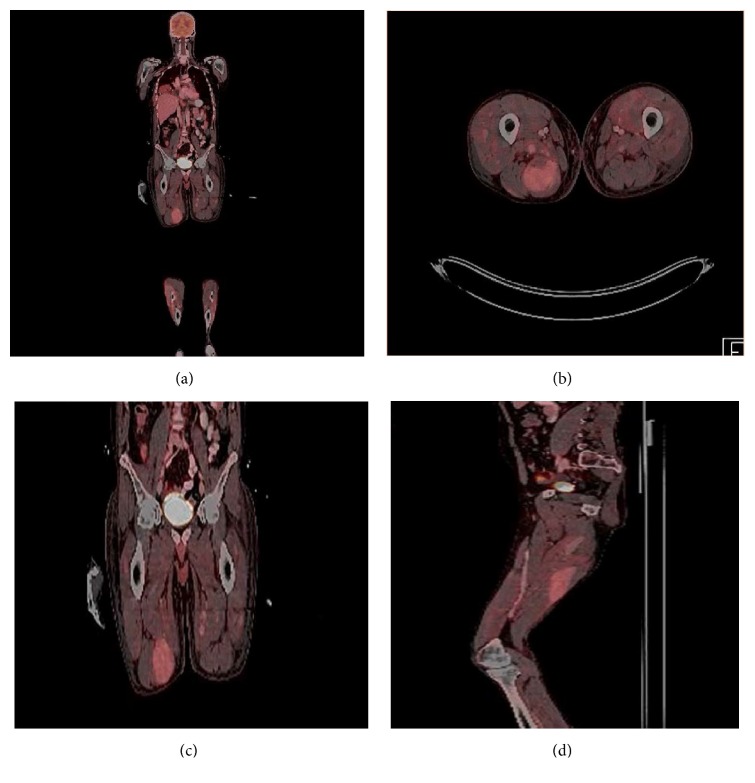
PET CT of the same 51-year-old male after approximately one week from the earlier shown US. ((a) and (c)) are coronal images of the whole body (a) and thighs (c) showing multiple foci of increased FDG uptake. (b) is an axial image of the distal thighs showing multiple foci of increased FDG uptake; the largest lesion on the posterior right thigh has SUV max of 4.3. (d) is a sagittal view of the same lesion showing its craniocaudal extension. Lesions were initially presumed and later biopsy proven to represent extra-axial multiple myeloma.
